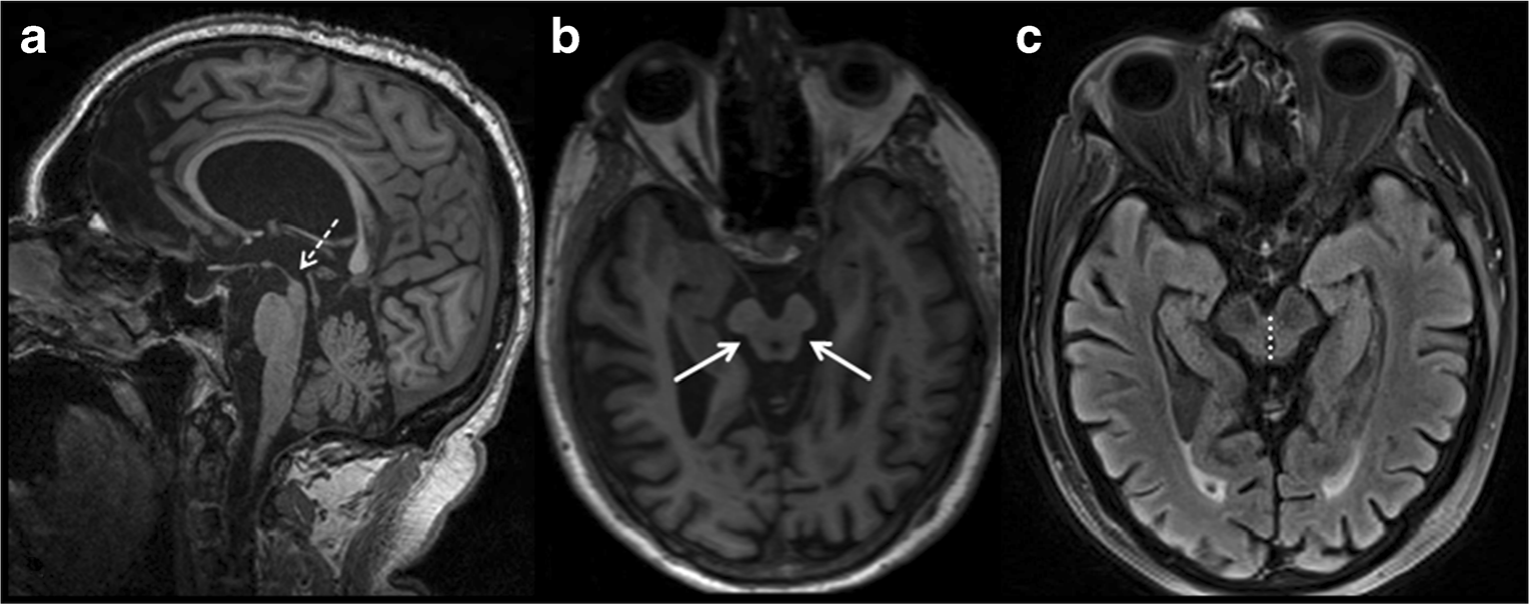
Progressive Supranuclear Palsy (PSP). A 79-year-old male with gait ataxia and frequent falls with forgetfulness and loss of interest in routine activity. a sagittal T1W image of the brain shows loss of superior convexity of midbrain: “Humming bird or penguin sign” (*white dashed arrow*). b axial T1W section of the brain at the level of midbrain reveals abnormal concavity of lateral margin of midbrain: “morning glory sign” (*white arrows*). c axial FLAIR imaging of brain is demonstrating decreased anteroposterior diameter of midbrain (*white dashed arrow*)
